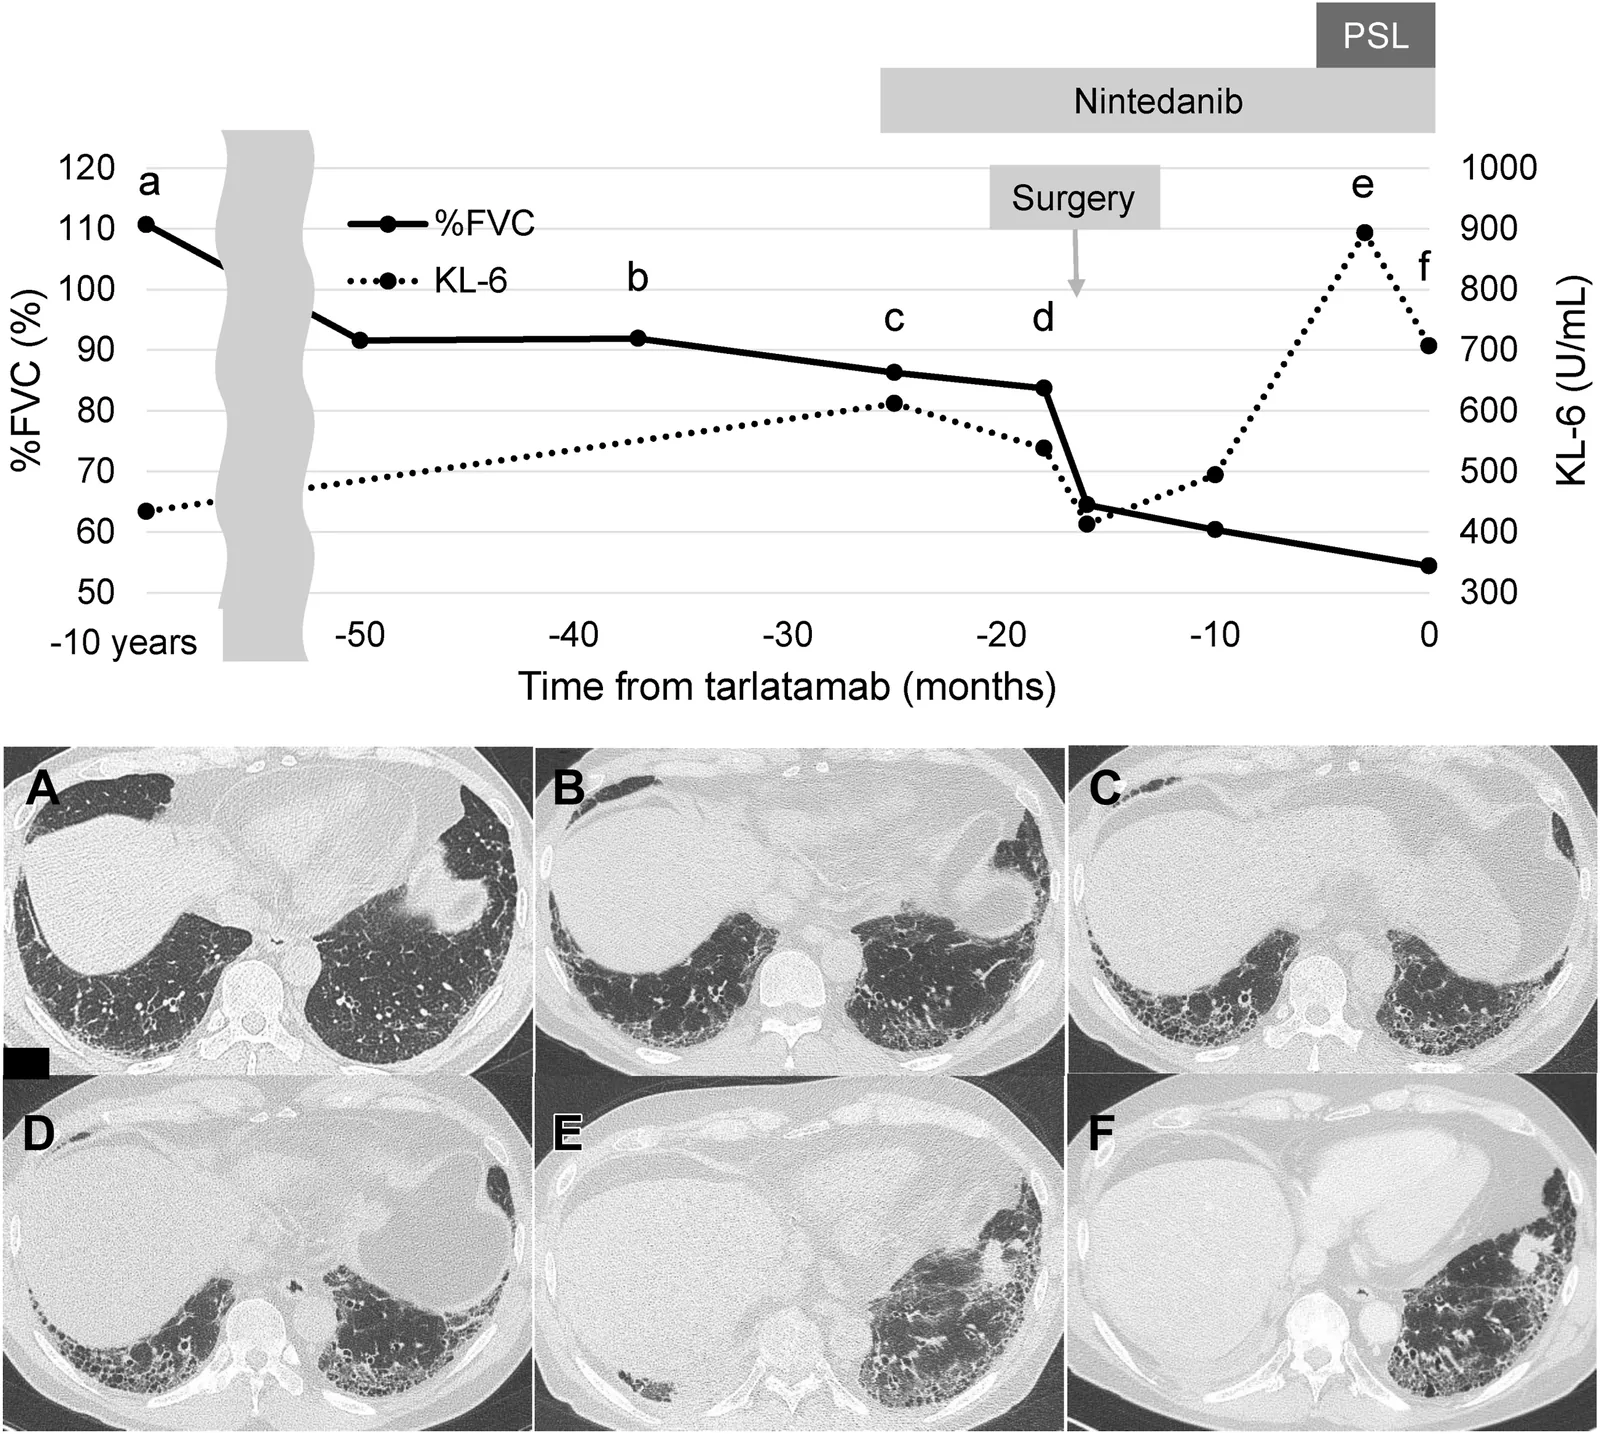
Clinical course of idiopathic pulmonary fibrosis before tarlatamab treatment. Chest HRCT obtained approximately 10 years before tarlatamab revealed subpleural reticulation in the lower lobes (*A*). Fibrosis and pulmonary function gradually worsened (*B*: 37 mo and *C*: 25 mo before tarlatamab). After nintedanib was initiated, disease progression was suppressed (*D*: 18 mo before tarlatamab administration). The patient subsequently underwent right lower lobectomy and was diagnosed with limited-stage SCLC (pT1cN2aM0, stage IIB). Acute exacerbation of IPF occurred 3 months before tarlatamab, with new ground-glass opacities and a decline in pulmonary function (*E*), which improved after corticosteroid treatment (*F*). % FVC, percent-predicted forced vital capacity; HRCT, high-resolution computed tomography; IPF, idiopathic pulmonary fibrosis; KL-6, Krebs von den Lungen-6; PSL, prednisolone.
